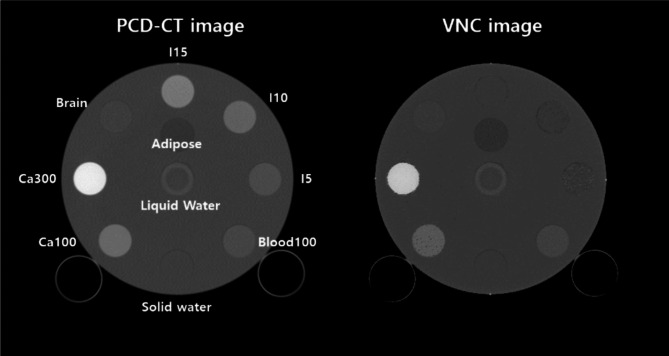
The PCD-CT image and its VNC image. The threshold for VNC was set as 0.6.
